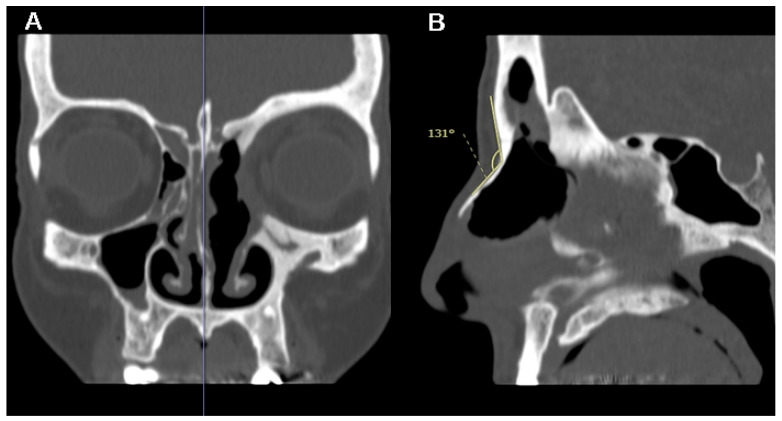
A patient with left fronto-ethmoidal sinusitis with orbital involvement undergoing a DRAF III surgery. On the coronal CT-scan, the mid-sagittal plane is identified by the location of nasal septum and crista galli (blue vertical line in (A)); the angle between the glabella and nasion (G-N angle) is then calculated on exactly these mid-sagittal sections (B).
